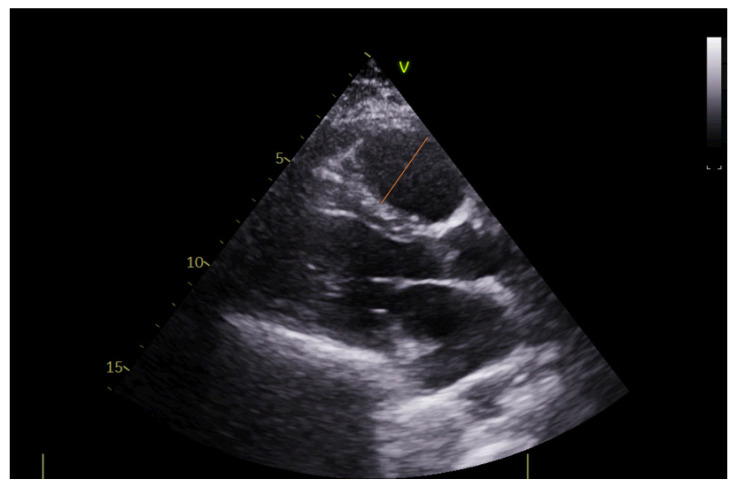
Right ventricular diameter from the parasternal long-axis (PLAX) view.
